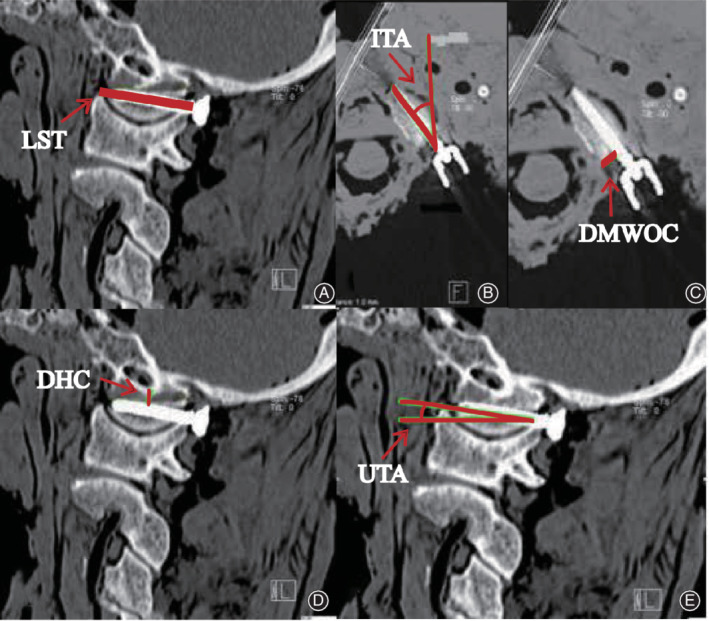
The measurements of (A) the length of screw trajectory (LST), (B) the inside tilting angle (ITA), (C) the distance to the medial wall of the OC (DMWOC), (D) the distance to hypoglossal canal (DHC) (D), and (E) the upper tilting angle (UTA). CT images show the different parameters for screw insertion.
